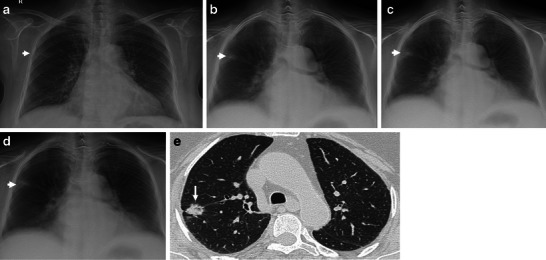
a-e A 60-year-old man with a suspected pulmonary lesion on the right lung. a Posteroanterior chest radiography in the upright position shows one suspected pulmonary nodule in the right lung (*arrow*). b-d Digital tomosynthesis images show the existence of a true lung opacity (*arrow*). e CT confirms the pulmonary opacity in the right lung (*arrow*)
